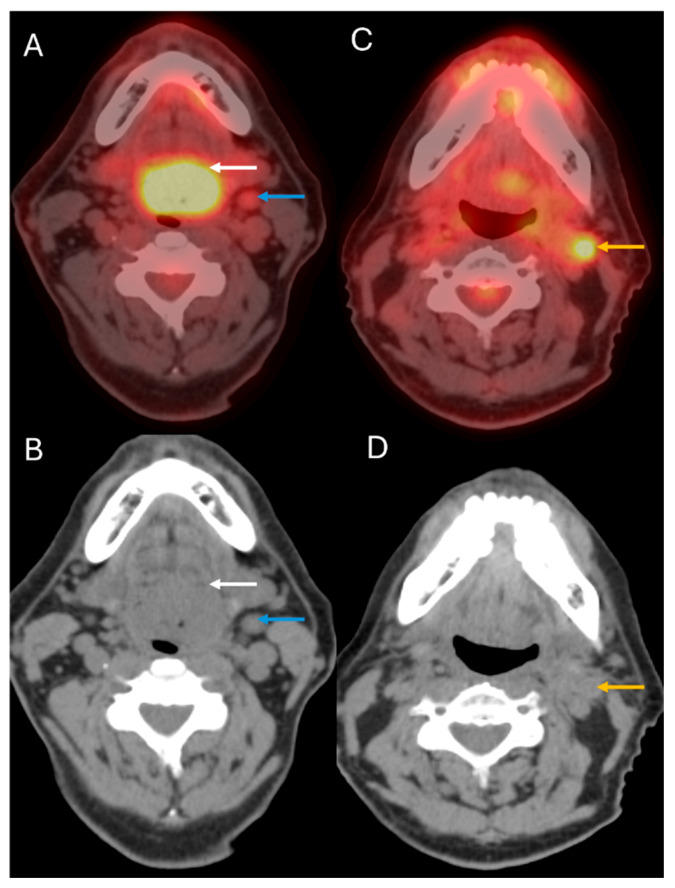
Recurrent disease detection: 63-year-old man with APV-negative SCC of the oropharynx treated with chemoradiation. Axial fused PET/CT (A) and axial CT (B) of the staging 18F-FDG PET/CT demonstrate the primary tumor (white arrows) and left cervical nodal metastasis (blue arrows). Axial fused PET/CT (C) and axial CT (D) images of the restaging PET/CT performed approximately 5 years after treatment completion for a palpable neck node demonstrate recurrent left cervical nodal disease (yellow arrows).
